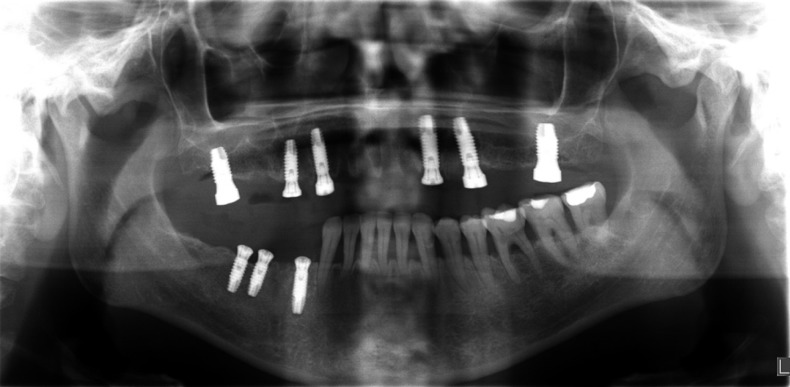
Panoramic radiography after implant placement.
